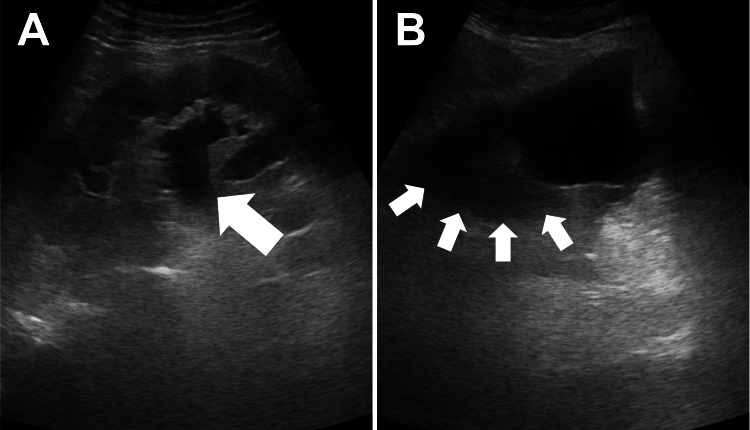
Ultrasound images obtained one month after RaRP with PLND (A) Significant right hydronephrosis was found (white arrow). (B) A hypoechoic peri-vesical area (white arrows) suggests a fluid collection, raising suspicion of a lymphocele. RaRP: robotic-assisted radical prostatectomy; PLND: pelvic lymph node dissection
